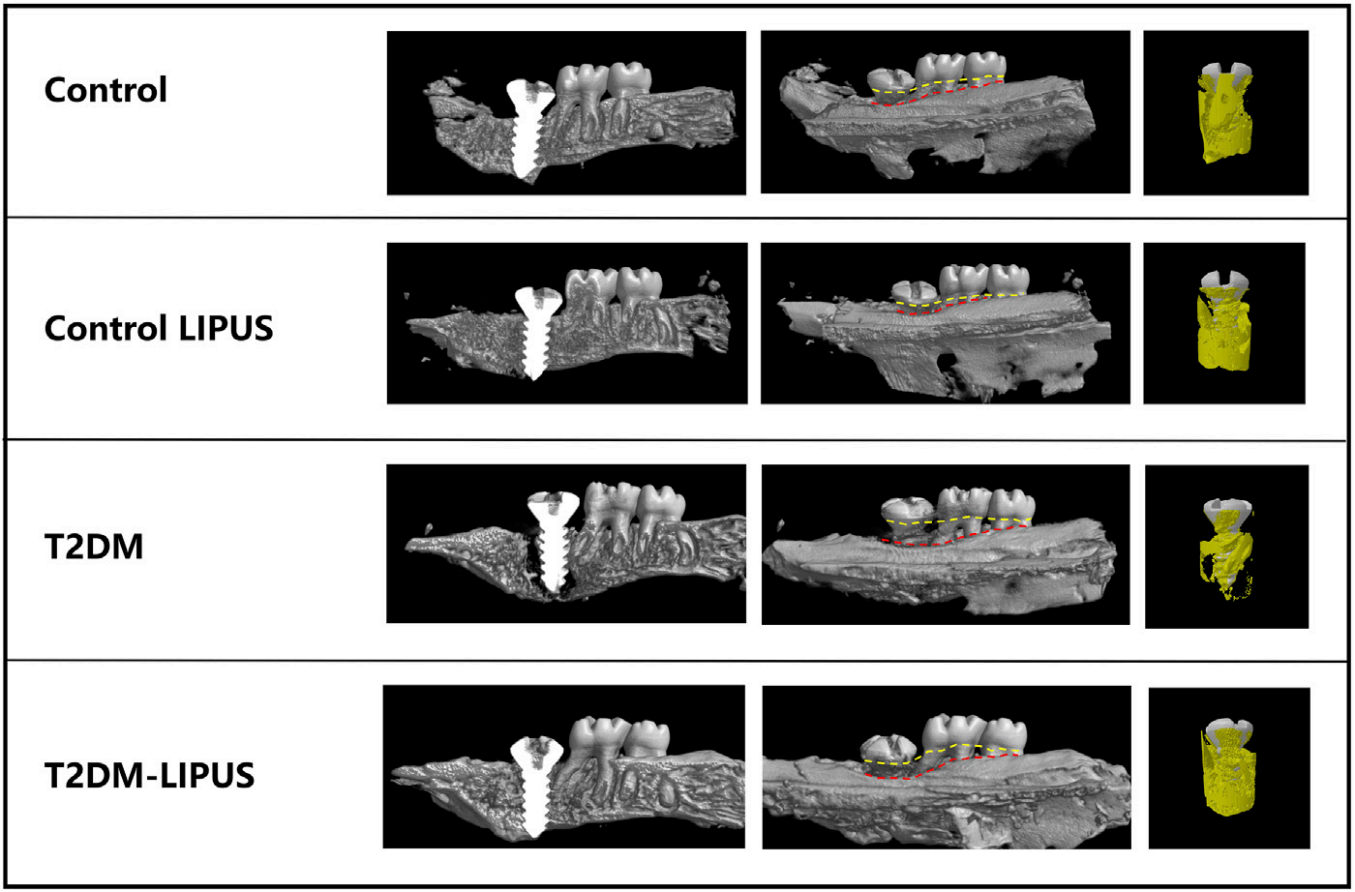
Three-dimensional visualization of the dental implant using Micro-CT technology. The marginal bone loss is assessed by the distance between the yellow and red line in the long axis direction of implants. Yellow line refers to the top of implant and cemento-enamel junction of the second and third molars. Red line refers to the marginal bone level. T2DM group showed significantly more marginal bone loss than other groups. T2DM, type II diabetes mellitus; LIPUS, low-intensity pulsed ultrasound.
